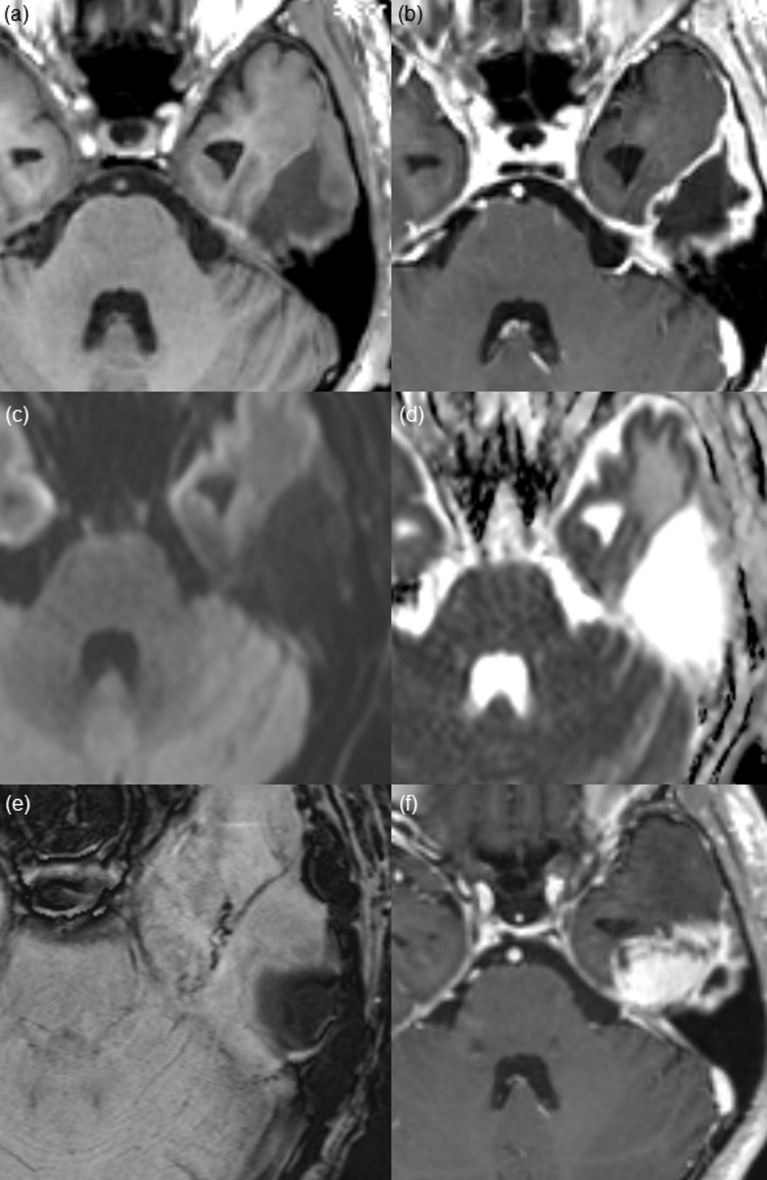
Thick wall enhancement pattern found on post-CCRT MR imaging from a 50-year-old male patient after gross total resection of glioblastoma. (a and b) Pre- and post-contrast enhanced T1 WI shows thick and irregular enhancement along the surgical cavity. (c) DWI and (d) ADC map show no diffusion restriction. Considering the dark signal intensity on (e) SWI, it reflects hemorrhage. (f) On 14-month follow-up contrast-enhanced T1 WI, the surgical cavity has shrunk, and a measurable mass has developed at the inferior aspect of the surgical cavity, which is pathologically confirmed as a recurred glioblastoma.
